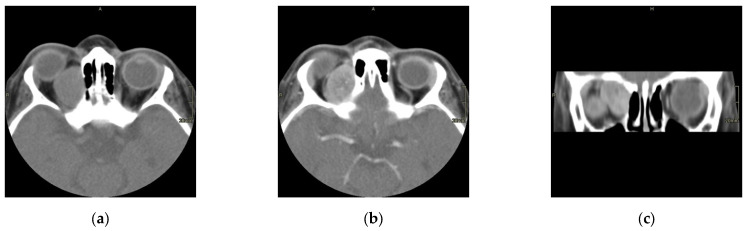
Computed scan tomography (CT) of orbital RMS case: non contrast axial section (a), contrast axial section (b), and contrast coronal section (c). This case shows a hypodense lesion on CT with post-contrast enhancement.
